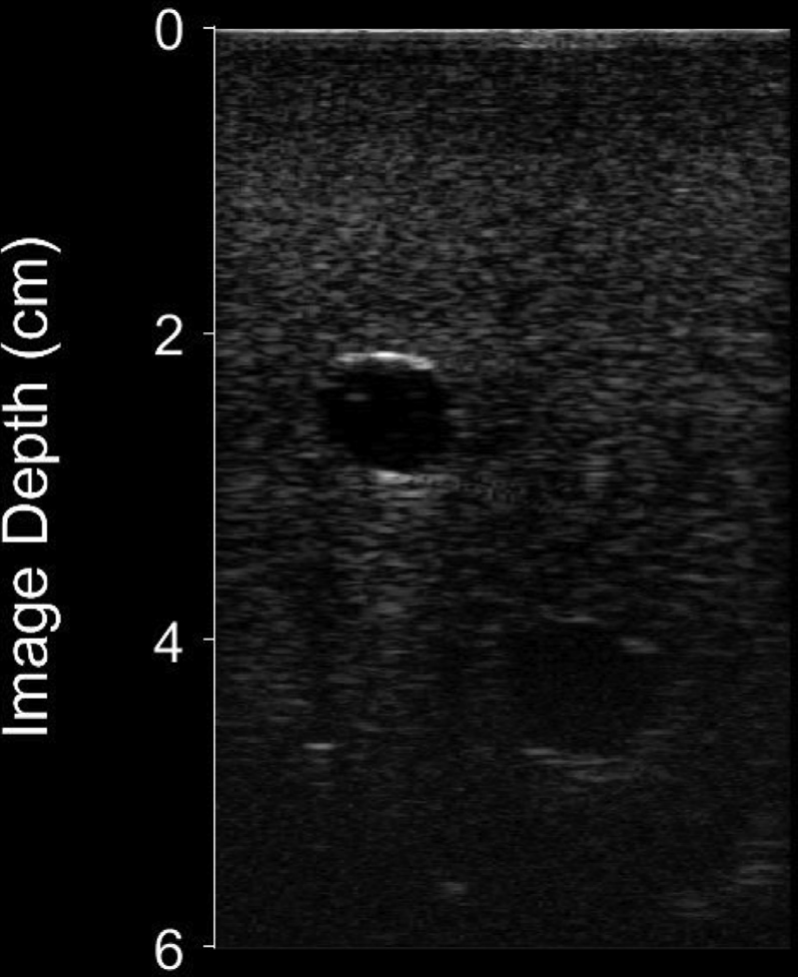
Static short-axis ultrasound image from the femoral vascular access model showing the right femoral artery (RFA) (left) and right femoral vein (RFV) (right). The RFV is notably 2 cm deeper than the RFA.
